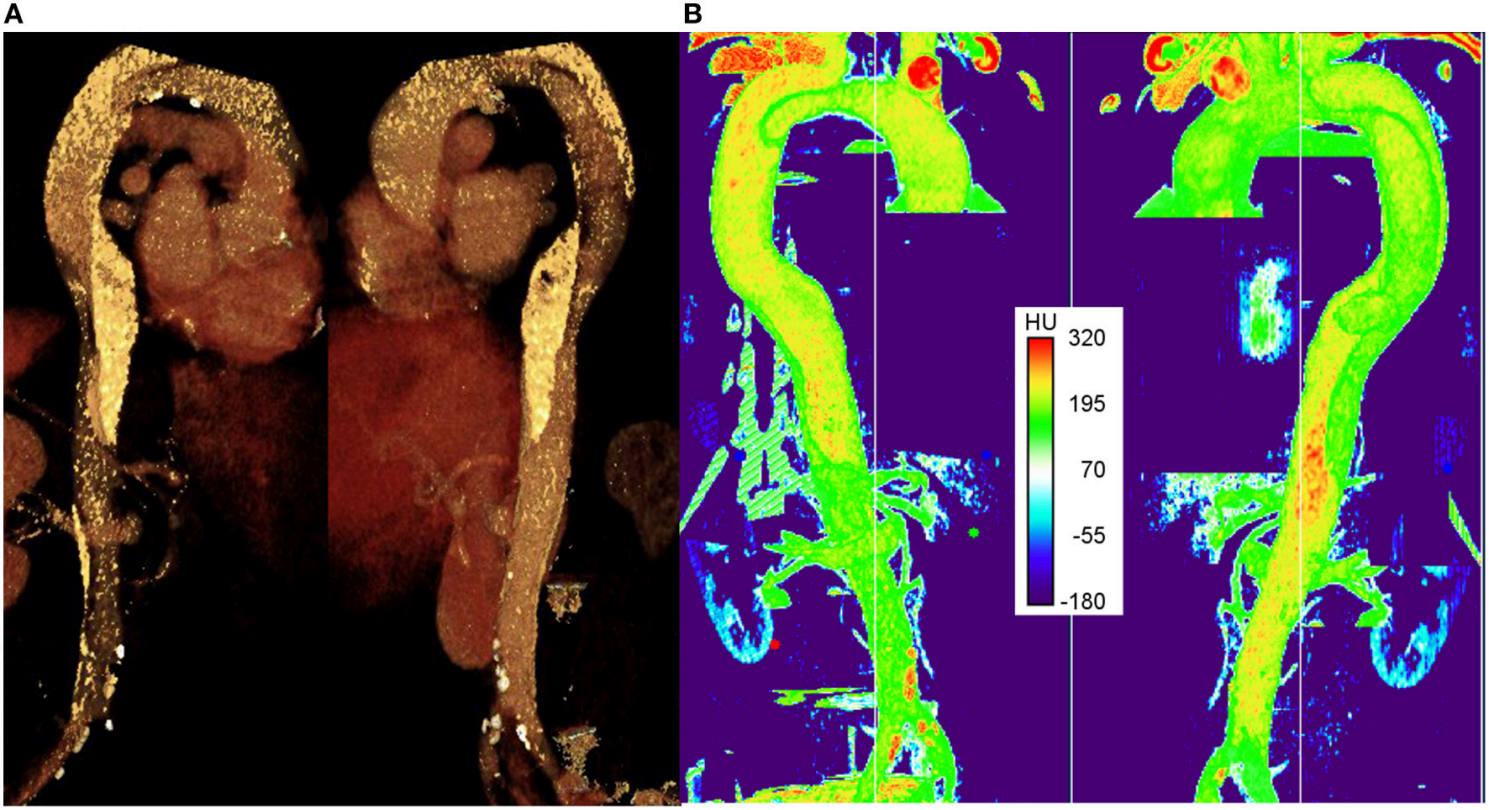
The right anterior and left posterior views of the aorta. (A) 3D CT data visualized in Aquarius and (B) 3D LUT volume viewer in FIJI (Image J).
